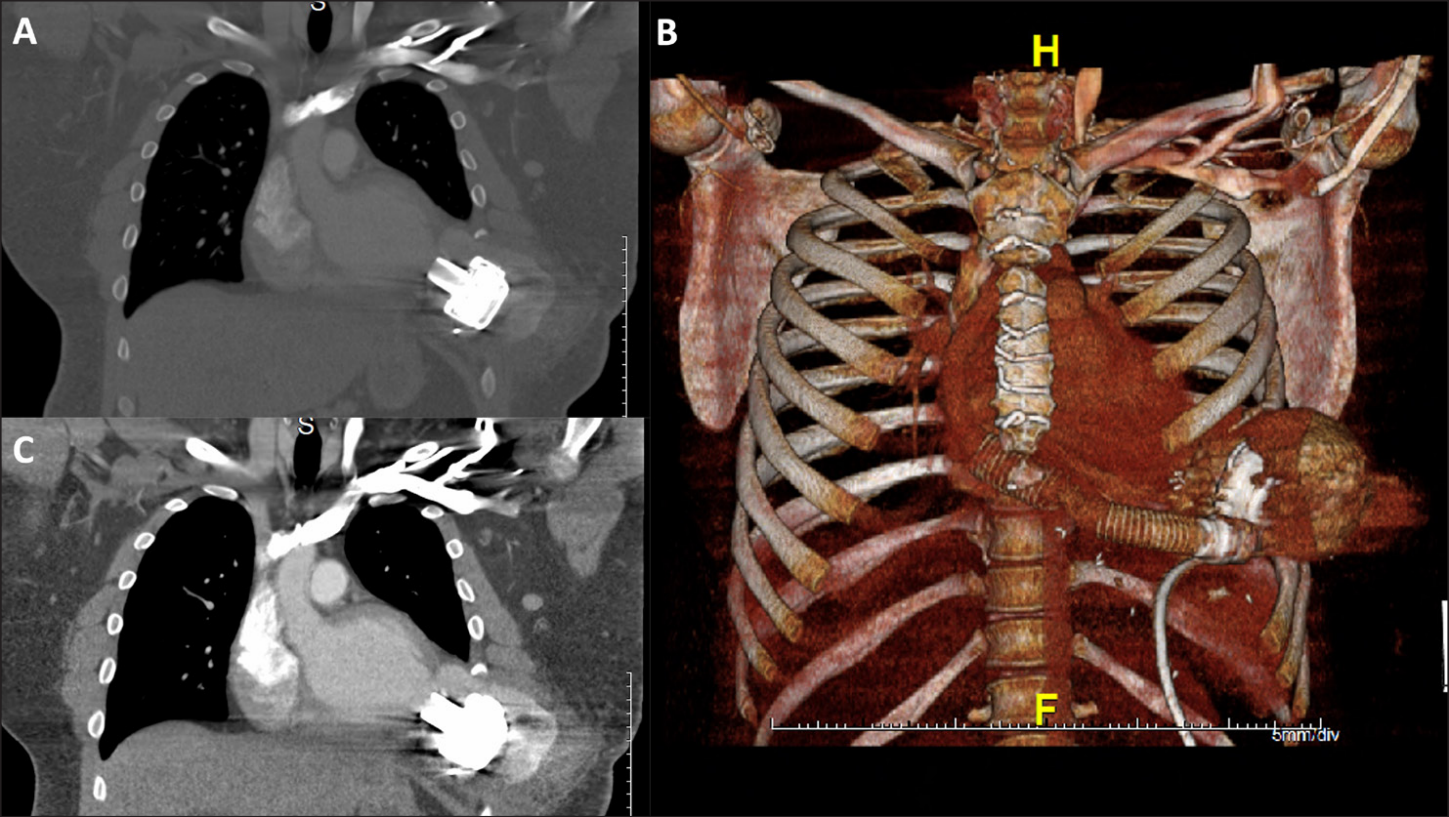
Computed tomography scan demonstrated a large left ventricular pseudoaneurysm measuring 10 × 10 × 8 cm in diameter surrounded by a hematoma. Coronal (A, C) and 3-dimensional reconstructed computed tomography (B) are shown. H: head; F: front
